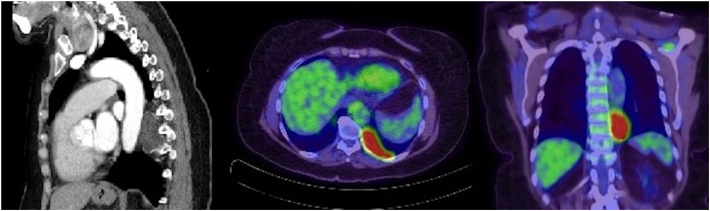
(Left) Sagittal contrast enhanced computed tomography chest scan demonstrating a paravertebral mass with prominent feeding vessels. (Middle, Right) Axial and Coronal images of a fused positron emission tomography/computed tomography scan demonstrating prominent 18F fluorodeoxyglucose uptake by the mass (maximum standardized uptake value, 13.8).
